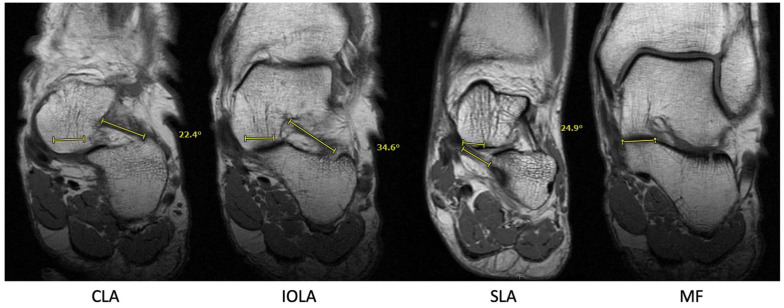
Coronal T1-weighted magnetic resonance images in a control patient demonstrating cervical ligament angle (CLA), interosseous talocalcaneal ligament angle (IOA), and spring ligament angle (SLA). All measurements were made with reference to a line drawn in parallel with the medial facet (MF).
